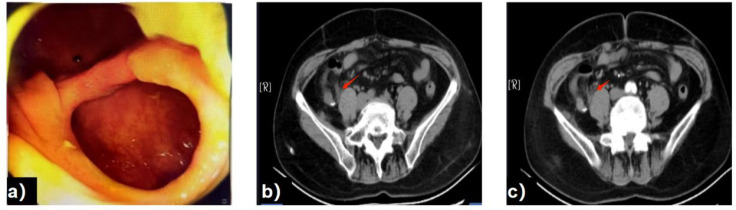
Colonoscopy (a) shows Colonic postoperative changes, no abnormalities identified. whole abdominal enhanced CT. (b, c) showed no signs of tumor recurrence.
